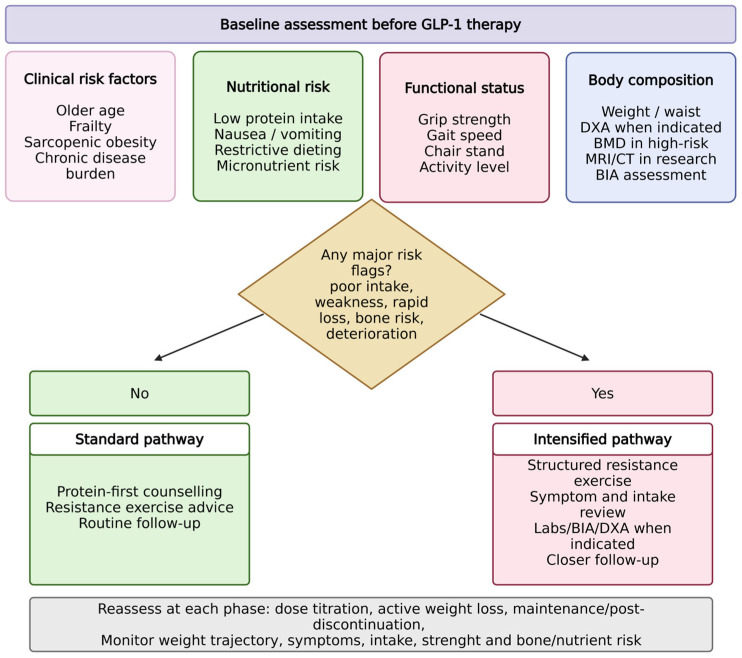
Pragmatic risk-stratification pathway for lean mass and musculoskeletal preservation during GLP-1-based obesity therapy. Baseline assessment integrates four domains: clinical risk factors, nutritional risk, functional status, and body composition. In patients without major risk flags, a standard pathway including protein-first counseling, resistance exercise advice, and routine follow-up is appropriate. Major risk flags, including poor intake, weakness, rapid weight loss, bone risk, or clinical deterioration, should prompt an intensified pathway with structured resistance exercise, symptom and intake review, laboratory and/or body composition reassessment when indicated, and closer follow-up. Risk status should be reassessed during dose titration, active weight loss, maintenance, and after treatment discontinuation, as nutritional intake, symptom burden, strength, and bone or nutrient risk may change over time. This framework is intended as a pragmatic clinical monitoring aid and should not be interpreted as a validated prediction score. BIA, bioelectrical impedance analysis; BMD, bone mineral density; CT, computed tomography; DXA, dual-energy X-ray absorptiometry; GLP-1, glucagon-like peptide-1; MRI, magnetic resonance imaging. Figure created with BioRender.com.
